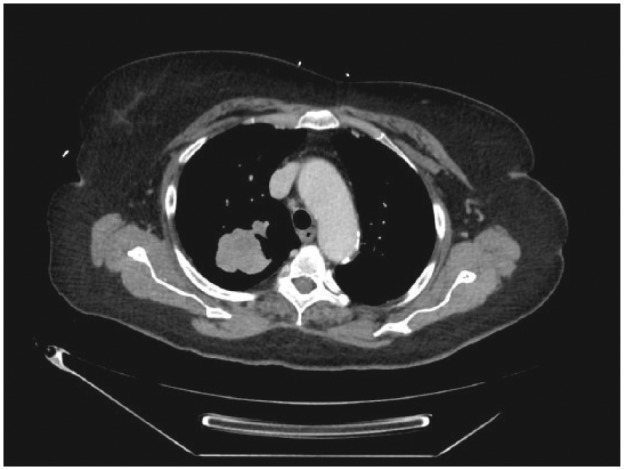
Computed tomography scan of the chest showing a right upper lobe lung mass.
